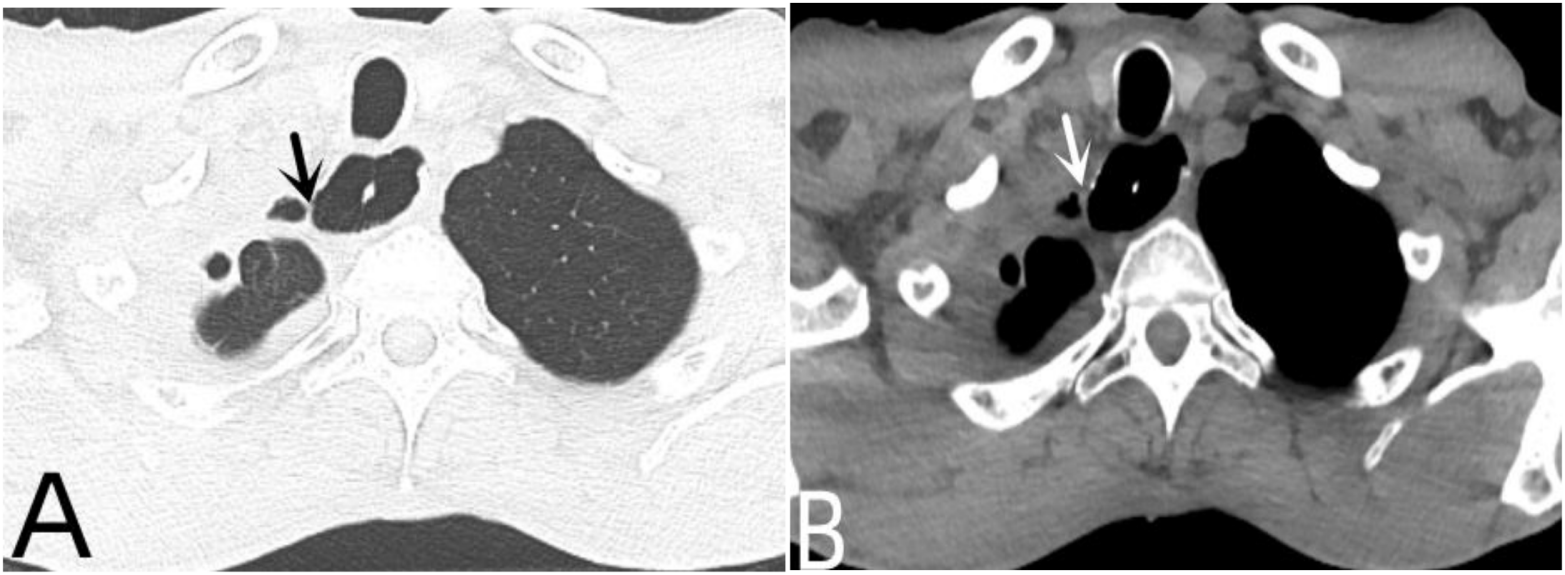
Showed the chest CT scan two weeks after discharge, in (A) (lung window) and (B) (mediastinal window), the arrows indicated the healed fistula.
